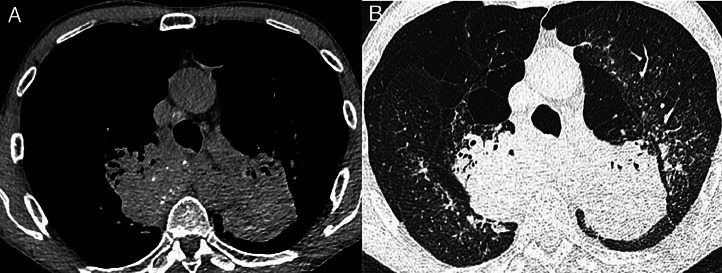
(A) Axial CT section (soft-tissue windows) showing complicated silicosis with progressive massive fibrosis. There are bilateral perihilar conglomerate masses with punctate calcification. Mediastinal calcified lymph nodes are also demonstrated. (B) Lung window settings at the same level show coexisting nodularity and emphysema.^7^
